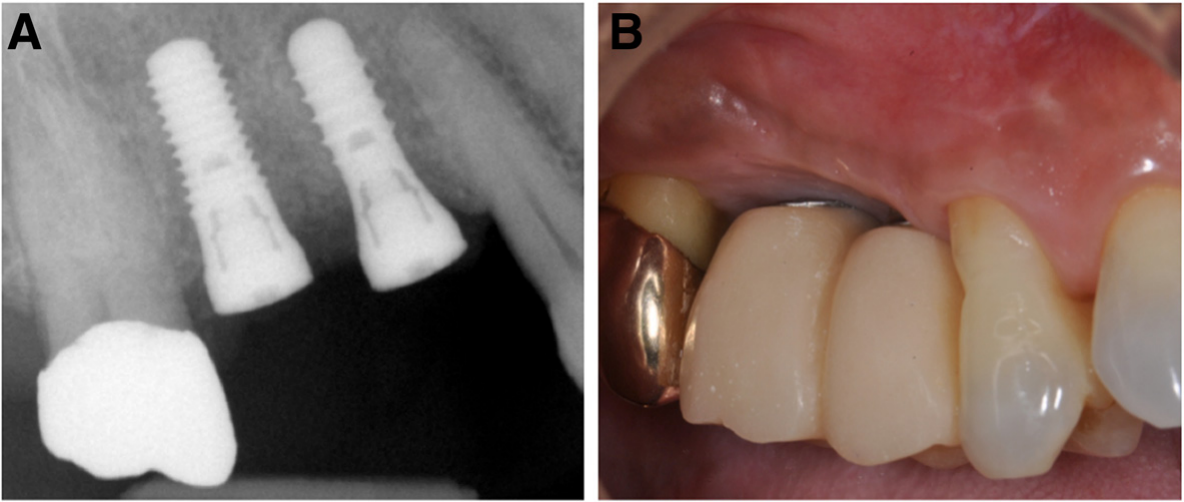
Preoperative dental X-ray (a) and preoperative intraoral view (b)
